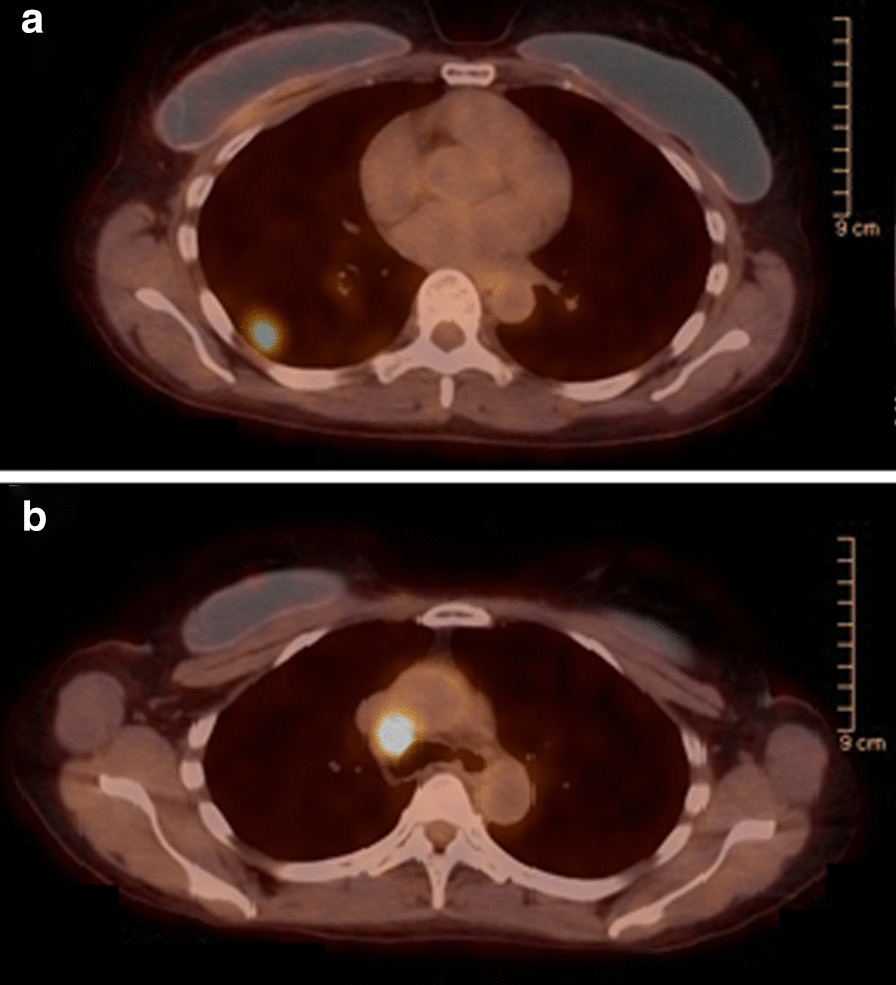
Disease status before and after neoadjuvant osimertinib therapy. Positron emission tomography/computed tomography at diagnosis. a Right lower lobe pulmonary nodule measuring 17 × 15 mm with maximum standardized uptake value (SUV_max_) of 7.6. b Prominent right paratracheal lymph node measuring up to 13 mm in the short axis with SUV_max_ of 12.9
